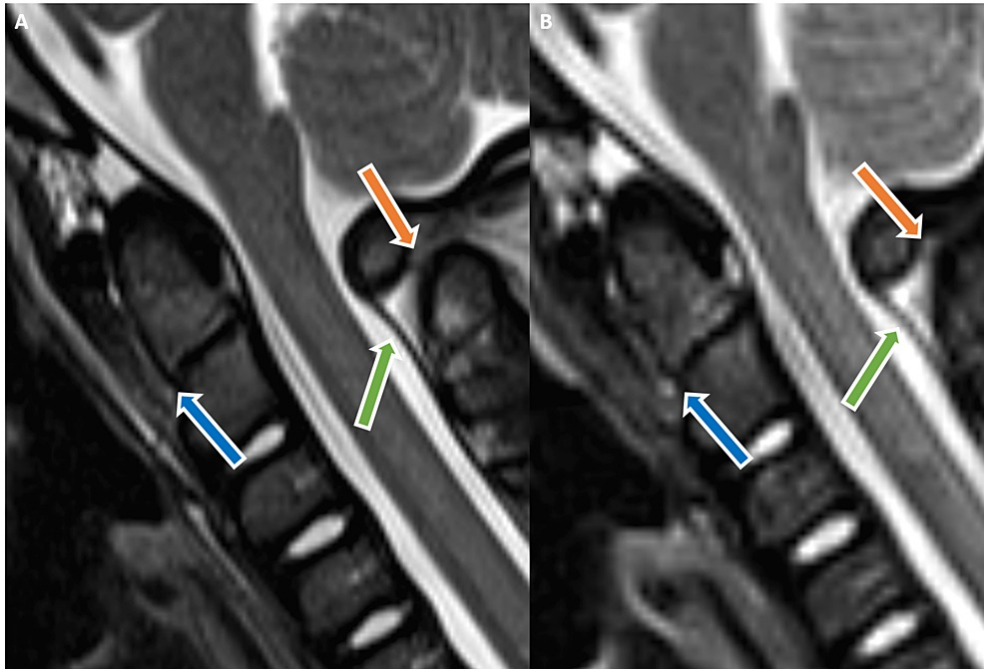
Sagittal T2-weighted (A) and STIR (B) MR images show abnormal hyperintensity with associated anterior tilting of the dens (blue arrows) consistent with odontocentral synchondrosal injury. Associated craniocervical junction posterior element injury involving the posterior atlantoaxial ligament (green arrows) and C1-C2 interspinous ligament (orange arrows) hyperintensity. STIR - short tau inversion recovery, MR - magnetic resonance
